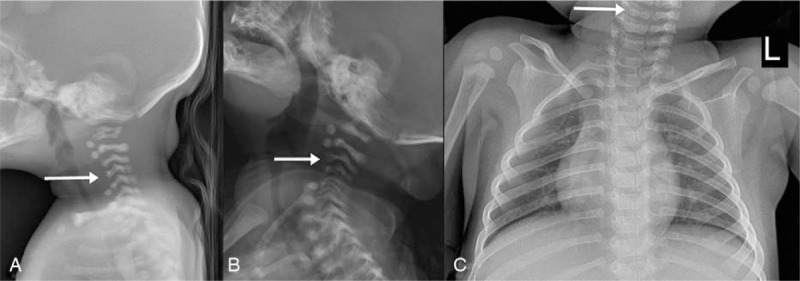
Female patient with Wolf-Hirschhorn syndrome. Lateral cervical spine and AP chest radiograph (A) at birth, (B) at 1 month of age, (C) at 13 months of age. The ossification centers (arrows) of C3 and C4 are invisible in (A) and (B), but visible in (C).
